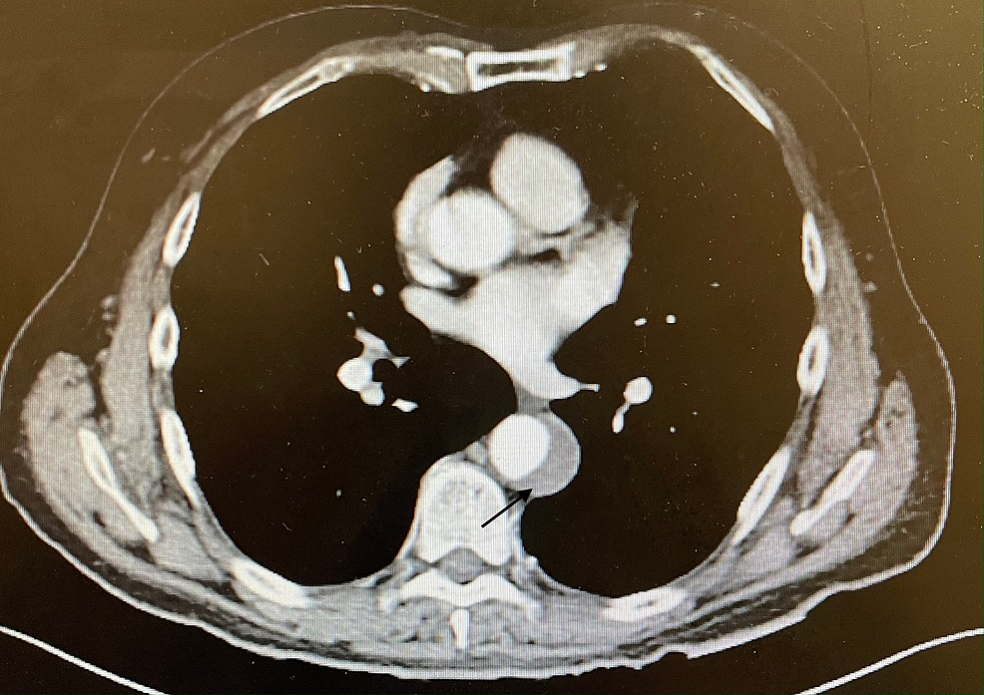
Focal arterial dissection along with thrombus extending upward in the descending thoracic aorta approaching the distal arch as seen on CT angiogram CT: computed tomography
